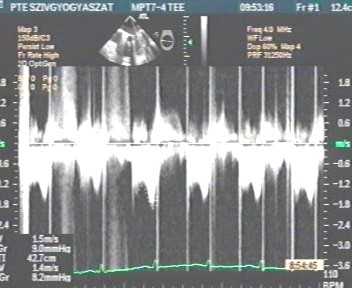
Transmitral continuous-wave Doppler showing abnormal hemodynamics of the prosthetic mitral valve. 2D-TEE confirmed the increase of the mean diastolic mitral gradient of 9 mmHg.
